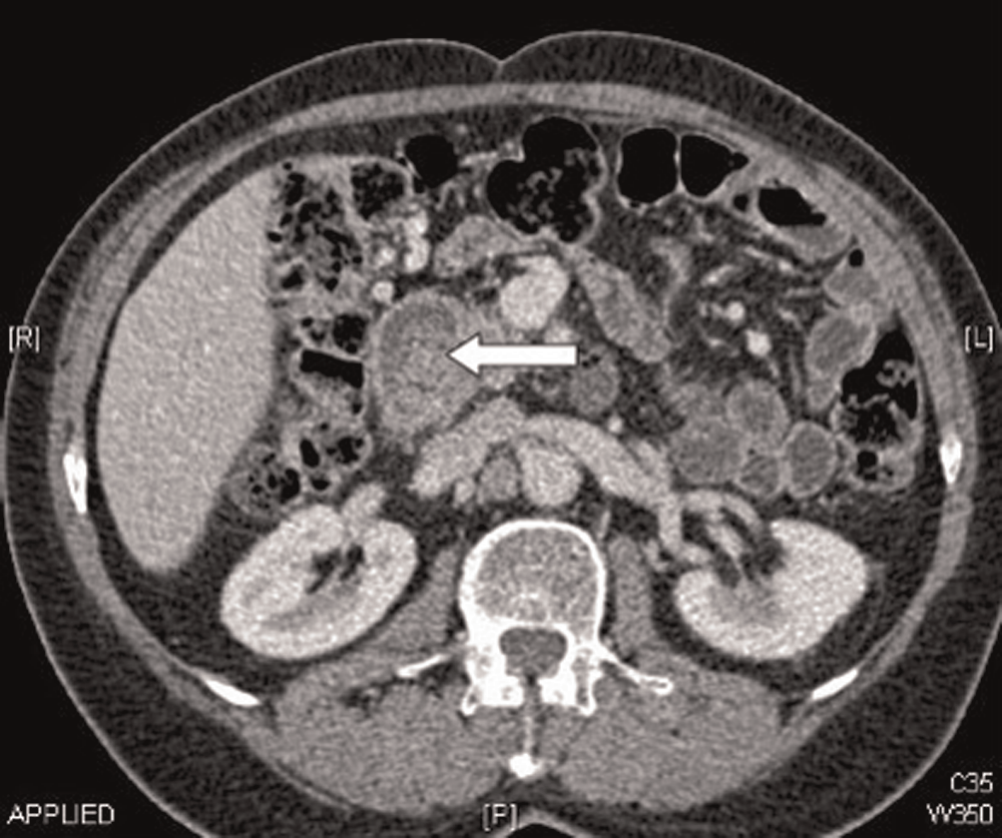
Computed tomography (b). Images showing a large filling defect in the duodenal stump, which had caused duodenal stump obstruction and extrinsic bile duct compression.
